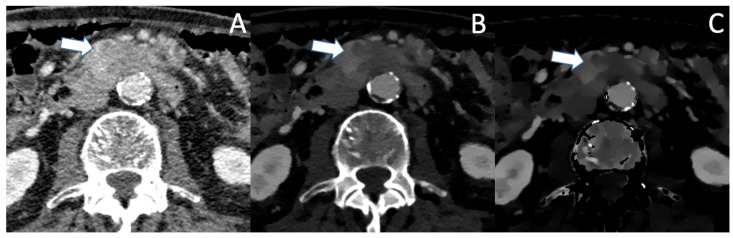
(A) The conventional CT image shows an ill-defined, moderately hypoattenuating mass of the pancreatic corpus in the portal venous phase (marked with the arrow). In VMI (B) and iodine density maps (C), the hypoattenuating tumor is more clearly visible with sharp demarcation to the adjacent pancreatic parenchyma.
